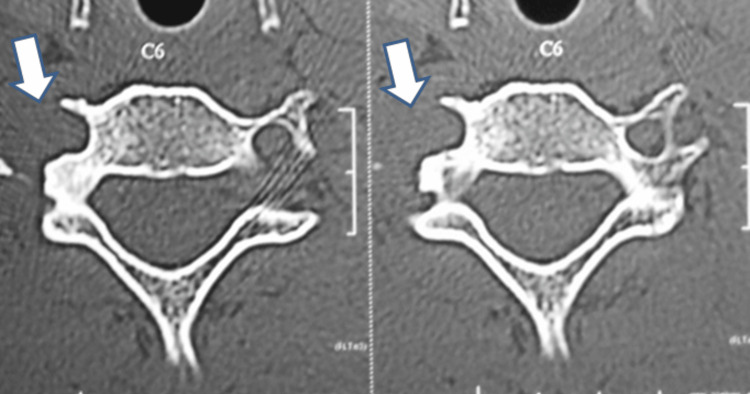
Postoperative axial CT scan at five-year follow-up showing total resection of the cervical osteoblastoma. Arrows indicate the surgical site, with no evidence of tumor recurrence CT: computed tomography; C6: sixth cervical vertebra
